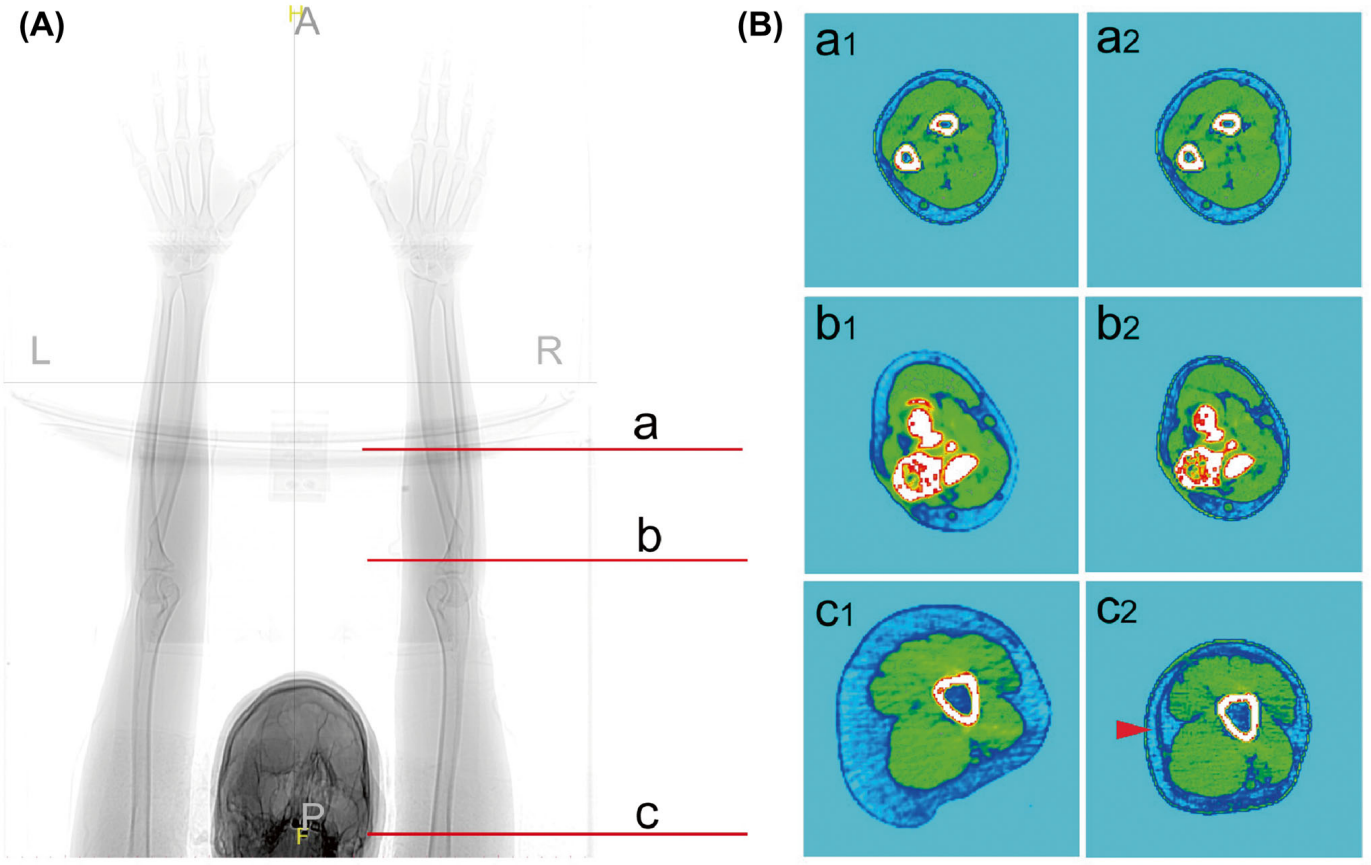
Comparison of pre and postoperative arm CT cross sections. Position selection and observation planes of plain CT scans of volunteers (a). Preoperative (B, a1–c1) and postoperative (B, a2–c2) images of different sections. Circular postoperative scar on the superficial fascia of the upper arm (red arrow in B, c2)
